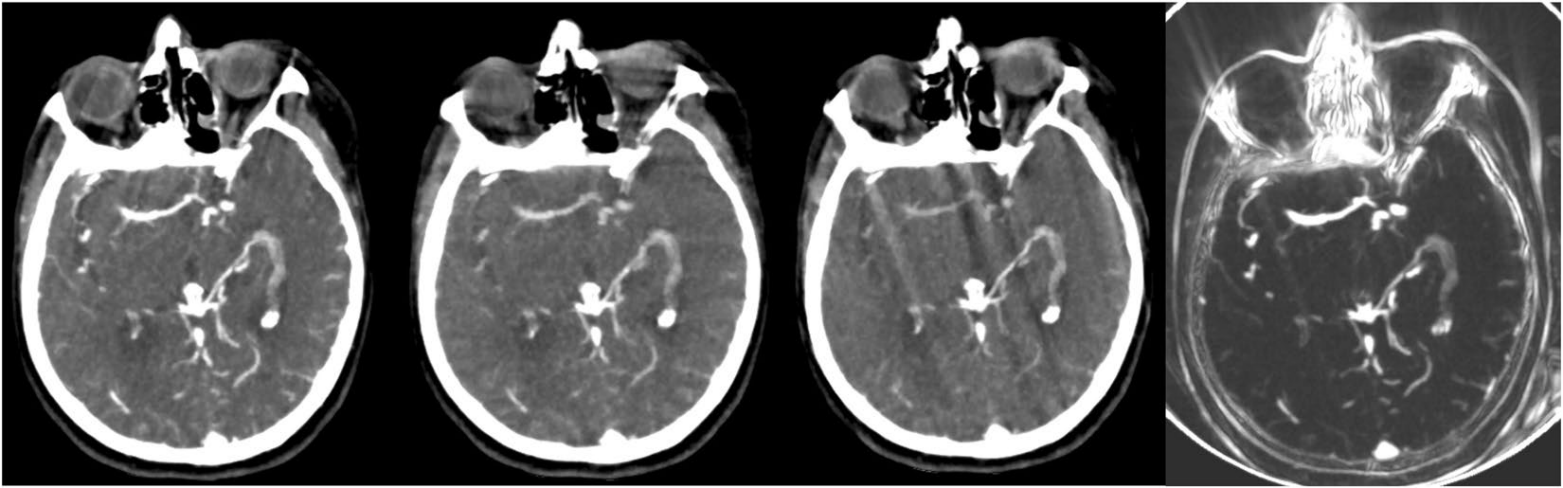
Axial view of three consecutive temporal acquisitions in a patient with beam hardening and motion artifacts in a single 4D CT acquisition and the resulting Weighted Temporal Variance (WTV) image (right).
